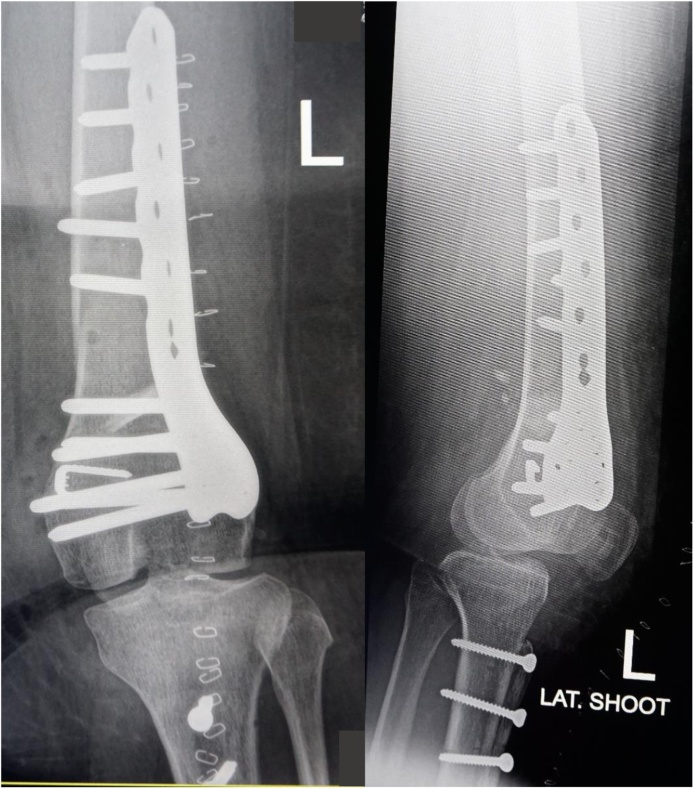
Skyline view radiograph show normal position of patella after reconstruction.
